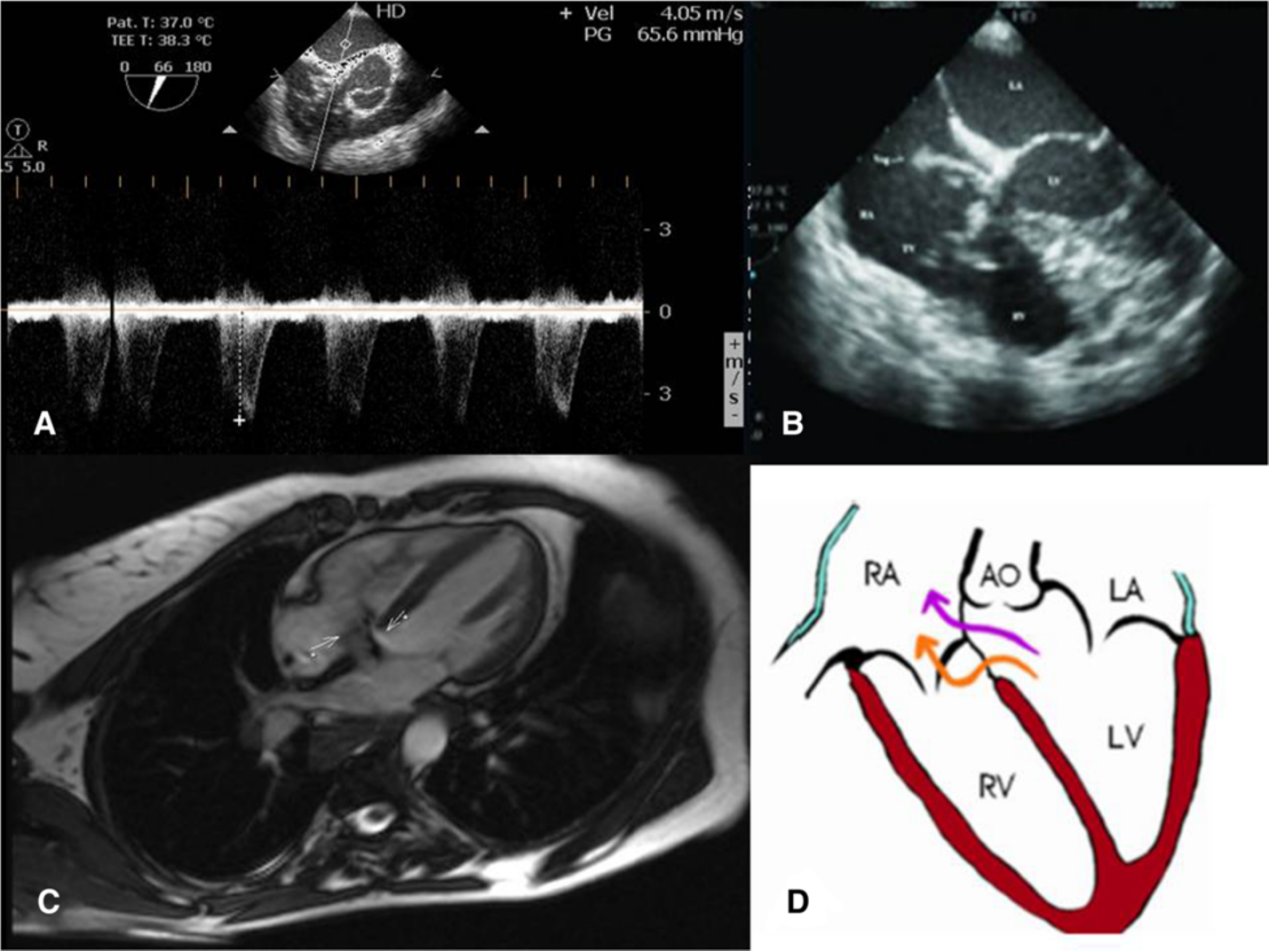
a Transesophageal echocardiography demonstrating the shunt between the left ventricle and right atrium. b Transthoracic echocardiography demonstrating the vegetation inserted above the septal leaflet of the tricuspid valve. c Cardiac magnetic resonance demonstrating a communication between the left ventricle and right atrium and right ventricle according to (d). C-type acquired Gerbode defect representing a supravalvular combined with n infravalvular communication between the left and right side of the heart
